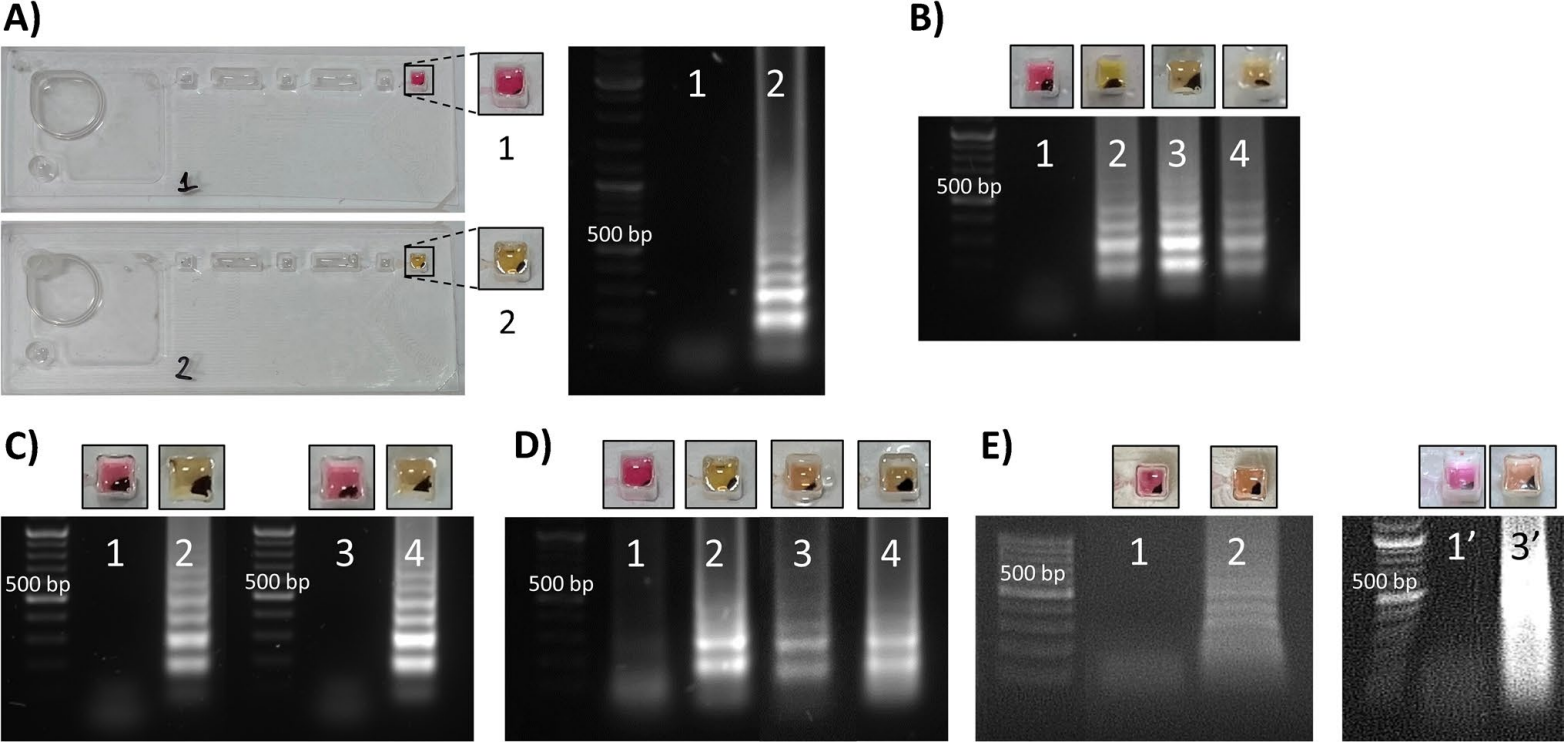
On-chip integrated steps of extraction and colorimetric LAMP detection of *N. gonorrhoeae* (NG) DNA. A Proof-of-concept IFAST-LAMP devices: 1 = remaining pink with no amplification for a no template control, 2 = turning yellow and showing amplification for 5 × 10^4^ copies/mL. B Aqueous 5 M GuHCl with 0.005% Tween 20 matrix spiked with NG DNA: 1 = no template control; 2 = 5 × 10^4^ copies/mL; 3 = 5 × 10^3^ copies/mL; 4 = 500 copies/mL. Devices incubated at 65°C for 40 min (*n* = 3). C Aqueous 5 M GuHCl with 0.005% Tween 20 matrix spiked with mixture of DNAs: 1 = 1.12 ng CT DNA + 5 × 10^5^ TV copies + 4 × 10^5^ TP copies; 2 = 5 × 10^5^ NG copies + 1.12 ng CT DNA + 5 × 10^5^ TV copies + 4 × 10^5^ TP copies; 3 = 0.112 ng CT DNA, 5 × 10^4^ TV copies, 4 × 10^4^ TP copies; 4 = 5 × 10^4^ NG copies + 0.112 ng CT DNA, 5 × 10^4^ TV copies, 4 × 10^4^ TP copies. Devices incubated at 65°C for 40 min (*n* = 1). D Sigmatrix synthetic urine containing 5 M GuHCl and 0.005% Tween 20 spiked with NG DNA: 1 = no template control; 2 = 5 × 10^4^ copies/mL; 3 = 5 × 10^3^ copies/mL; 4 = 500 copies/mL. Devices incubated at 65°C for 40 min (*n* = 1). E Human urine containing 5 M GuHCl and 0.005% Tween 20 spiked with NG DNA: 1, 1′ = no template control; 2 = 5 × 10^4^ copies/mL; 3′ = 5 × 10^3^ copies/mL. Devices incubated at 65°C for 40 min (1, 2) or 60 min (1′, 3′), *n* = 1
